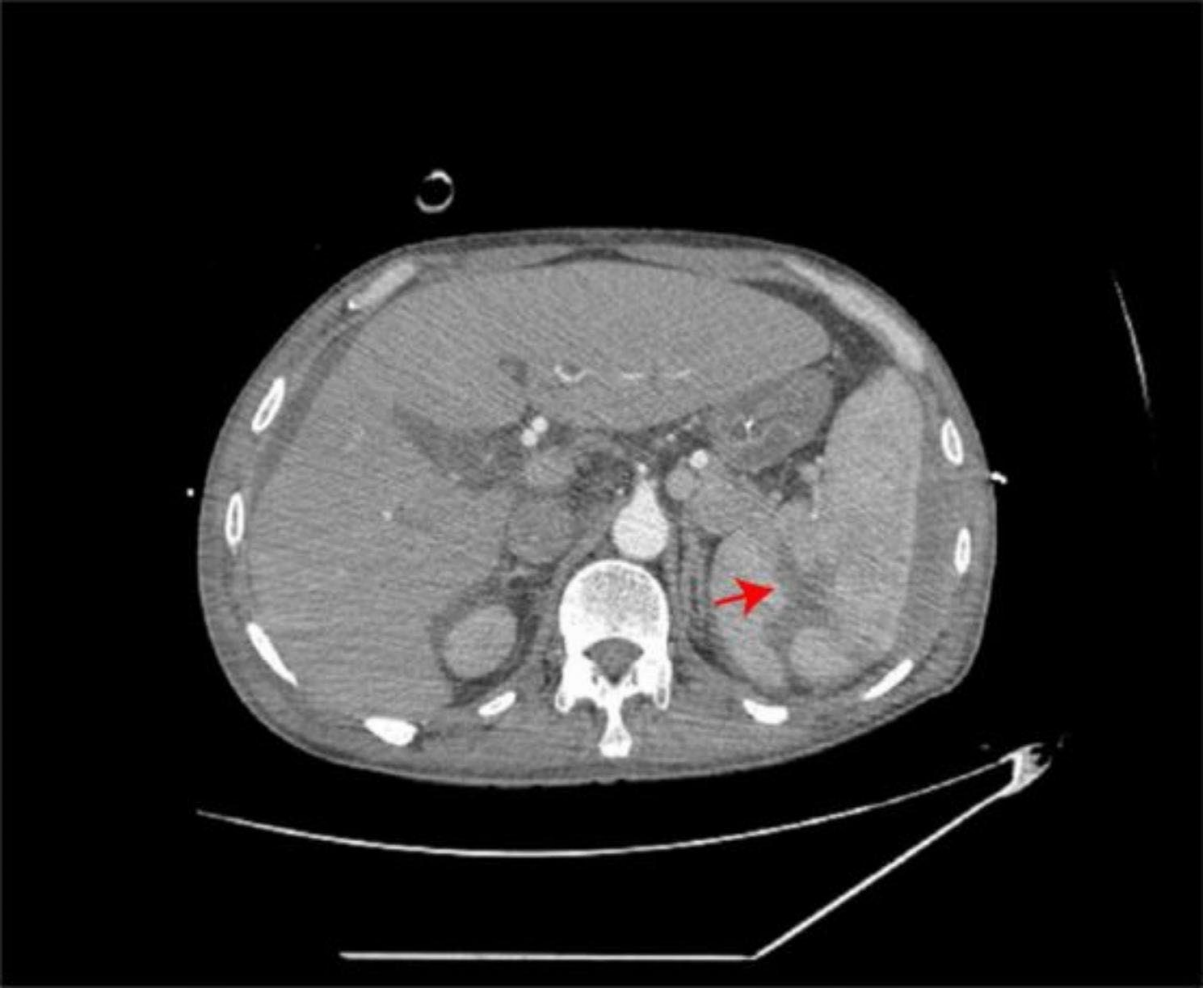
The abdomen-enhanced CT scan showed: a spleen laceration
